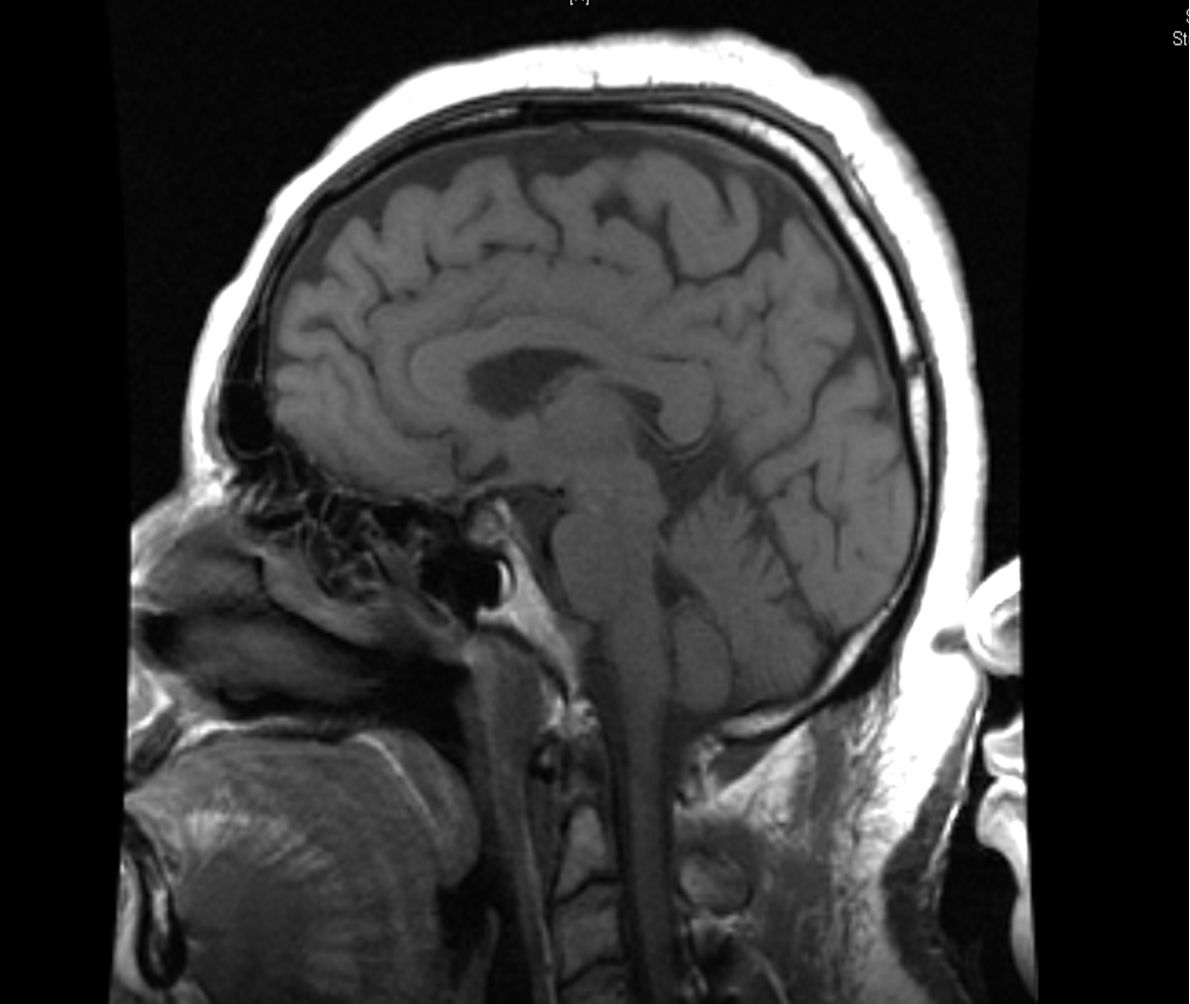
Normal post-operative sagittal T1-weighted MRI of patient's head.
